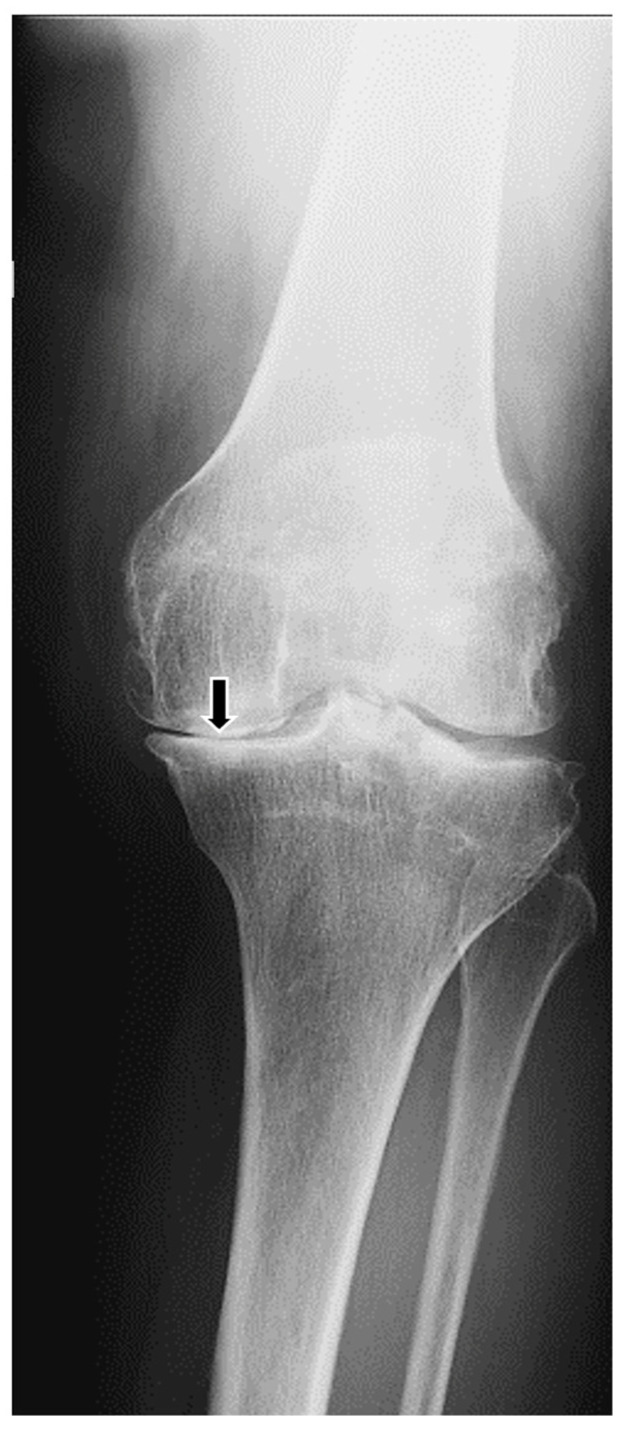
Anteroposterior radiograph of the patient’s knee taken 14 years ago when she was 69 years old. The medial tibiofemoral compartment articular cartilage has been destroyed (black arrow) and reactive bone and osteophytes have formed. Varus knee angulation has resulted. The radiographic appearance is typical of osteoarthritis which can result from a variety of chondral insults including genetic, inflammatory, septic, and mechanical conditions.
